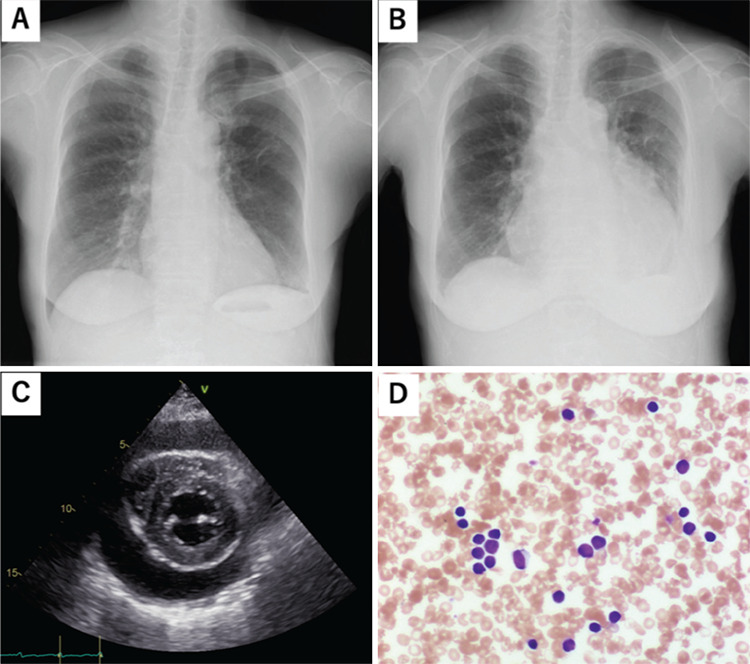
A, B) Chest X-ray revealed marked cardiomegaly (B), which was not apparent 2 months prior (A); C) transthoracic echocardiography showed a large pericardial effusion with right ventricular collapse; D) the drained bloody pericardial effusion contained many CLL cells. CLL: Chronic lymphocytic leukemia.
